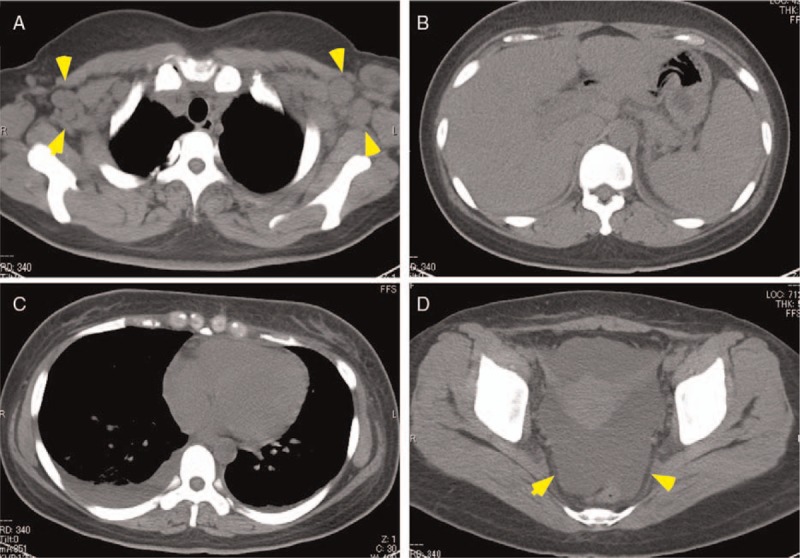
Computed tomography images obtained on admission, showing: (A) bilateral axillary lymphadenopathy (arrow heads), (B) bilateral pleural effusion, (C) hepatosplenomegaly, and (D) massive ascites (arrow heads).
